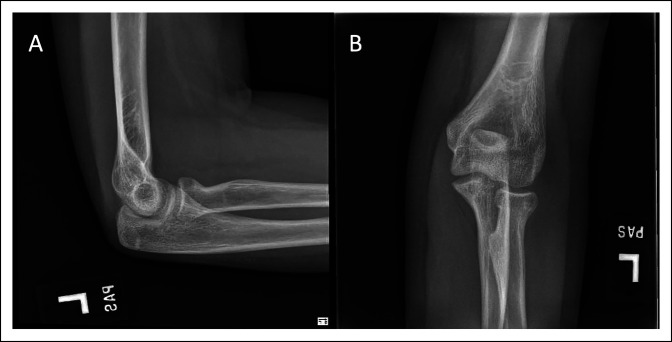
Images showing follow-up radiographs 3 months after fixation. Anteroposterior (A) and lateral (B) views of the left elbow after removal of the pins, with interval healing of the radial neck fracture and excellent radial neck, ulnohumeral, and radiodcapitellar alignment with no signs of displacement or osteonecrosis.
